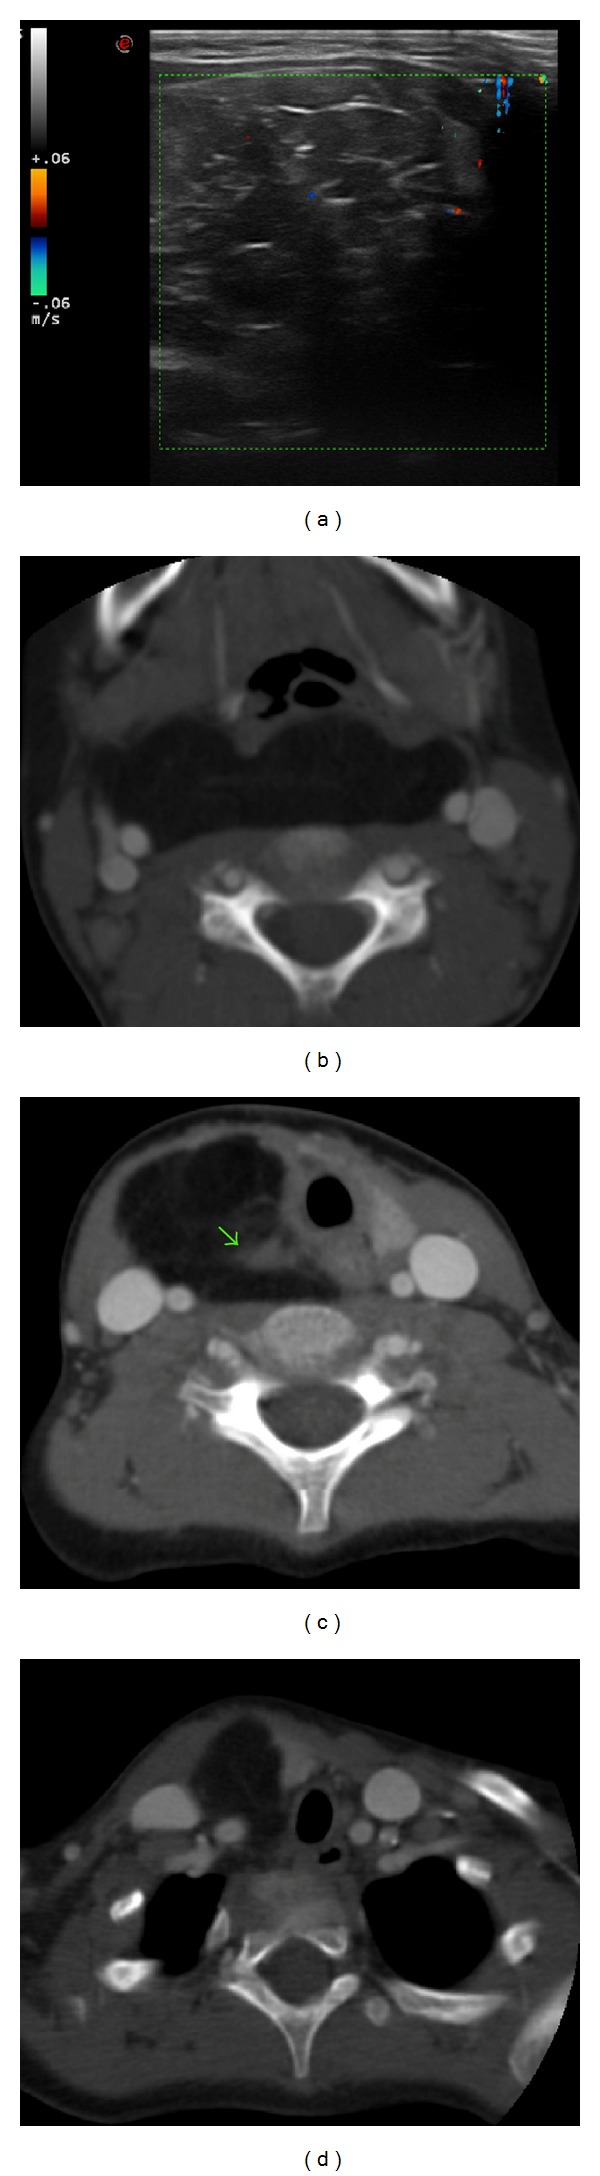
(a) US color-Doppler evaluation revealed low vascularity of the tumor. CT scan showed the extent of the fatty mass in (b) retropharyngeal space displacing the right vascular bound, (c) along the right side of the trachea, where along the right side of the trachea where an enhanced solid nodule (arrow) was appreciable an enhanced solid nodule (arrow), and (d) up to right supraclavicular fossa.
